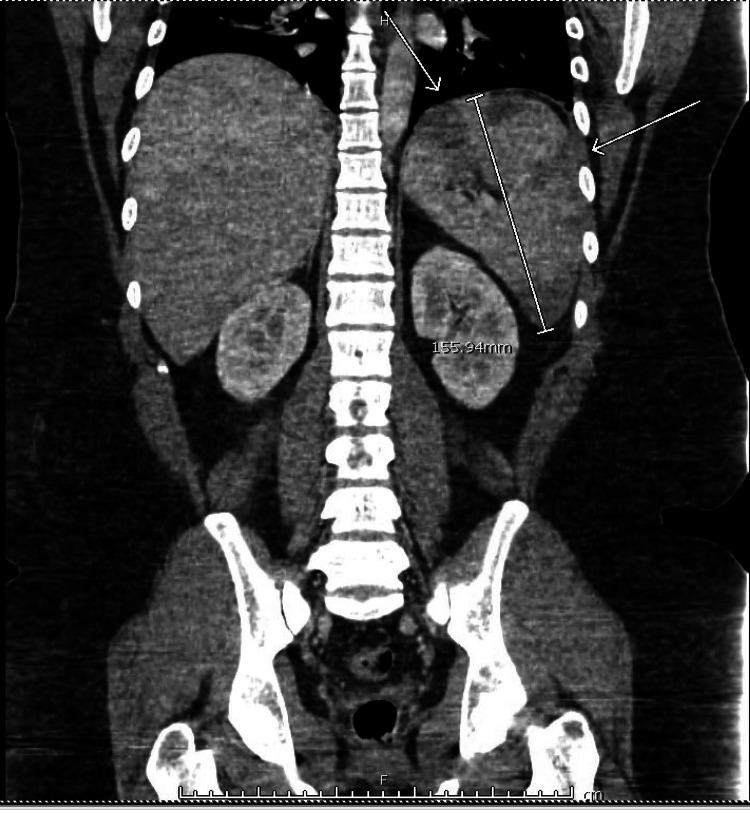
Coronal CT image shows a significantly enlarged spleen (white arrow) with multiple splenic infarcts.
